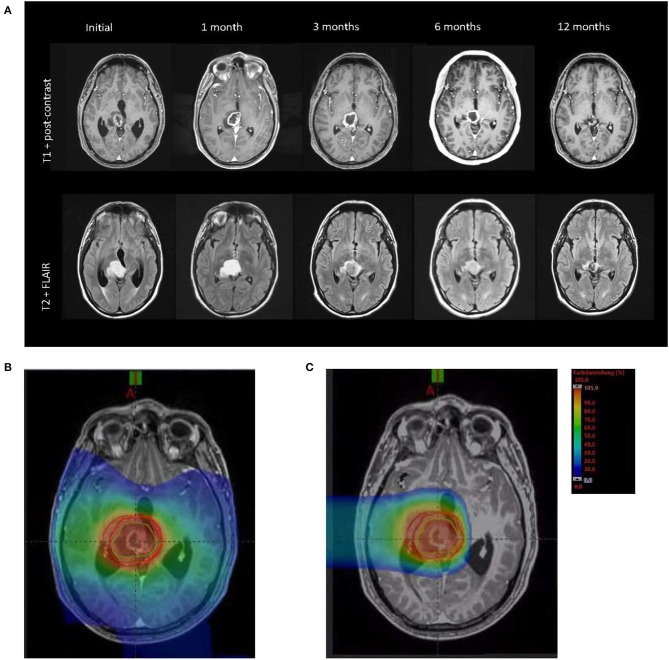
(A) MRI (T1 postcontrast and T2-FLAIR) showed pseudoprogression at 1 month after radiation therapy and complete radiological response after 1 year. (B) Radiation field photon therapy (25 × 2 Gy). (C) Radiation field proton therapy (5 × 2 Gy RBE).
